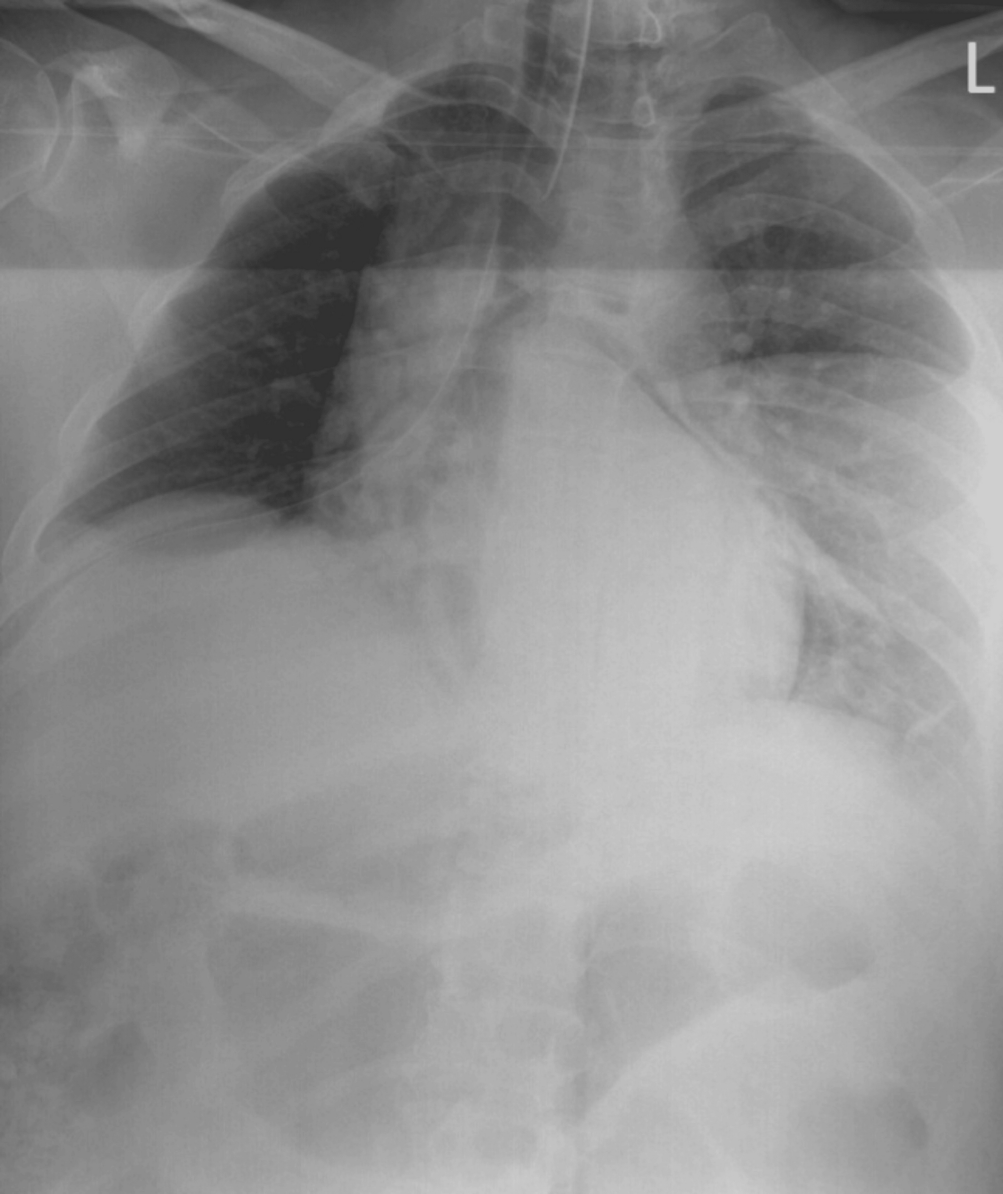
Chest X-ray obtained on postoperative day 0 X-ray completed in OR status post chest tube placement. The chest X-ray shows the tip of the right chest tube projects over the right upper mediastinum. The lungs are well expanded with asymmetric lung densities likely due to overlying soft tissue on the left hemithorax, due to patient rotation. There are bibasilar atelectatic changes noted. No definite pneumothorax is visualized.
